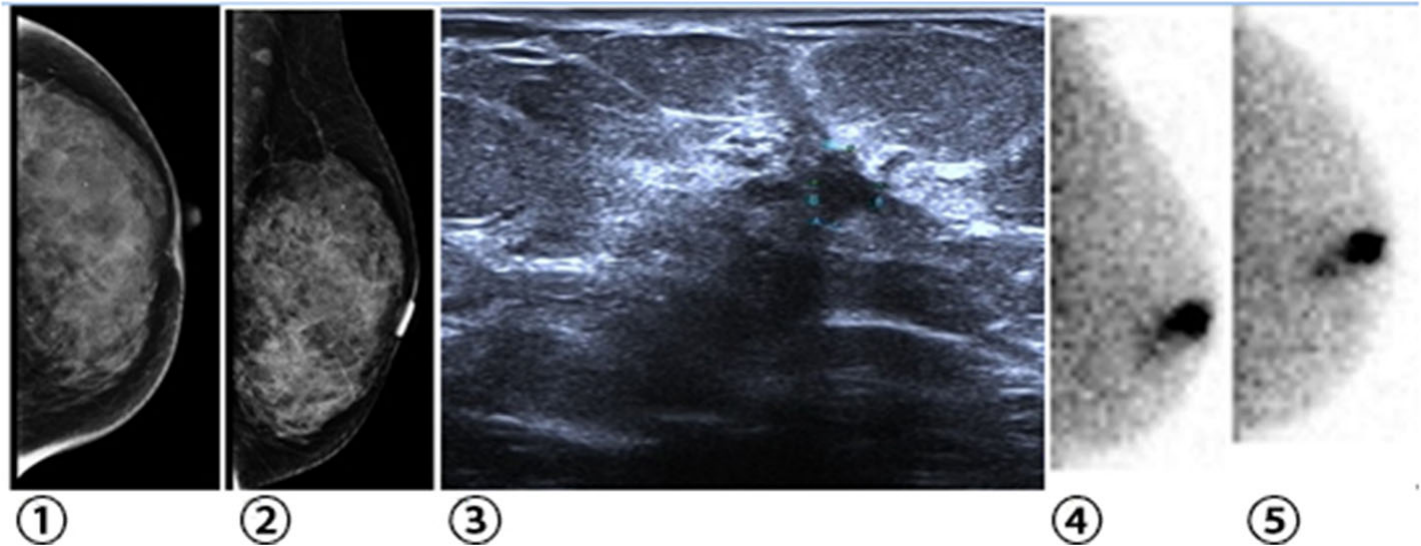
Breast images from a woman. (①②) No mammographic evidence of dense breast abnormalities; (③)An ill-defined hypoechoic nodule was detected via ultrasound in the left breast, 0.6 cm*0.5 cm, BI-RADS 4B; (④⑤) BSGI revealed a focal region of increased radiotracer uptake in the left breast, TNR = 3.21; Pathology confirmed the presence of a 7 mm invasive ductal carcinoma
